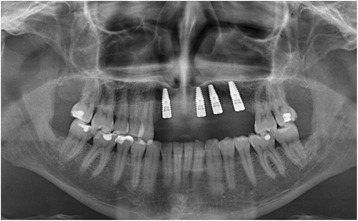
Orthopantomogram after implant insertion
